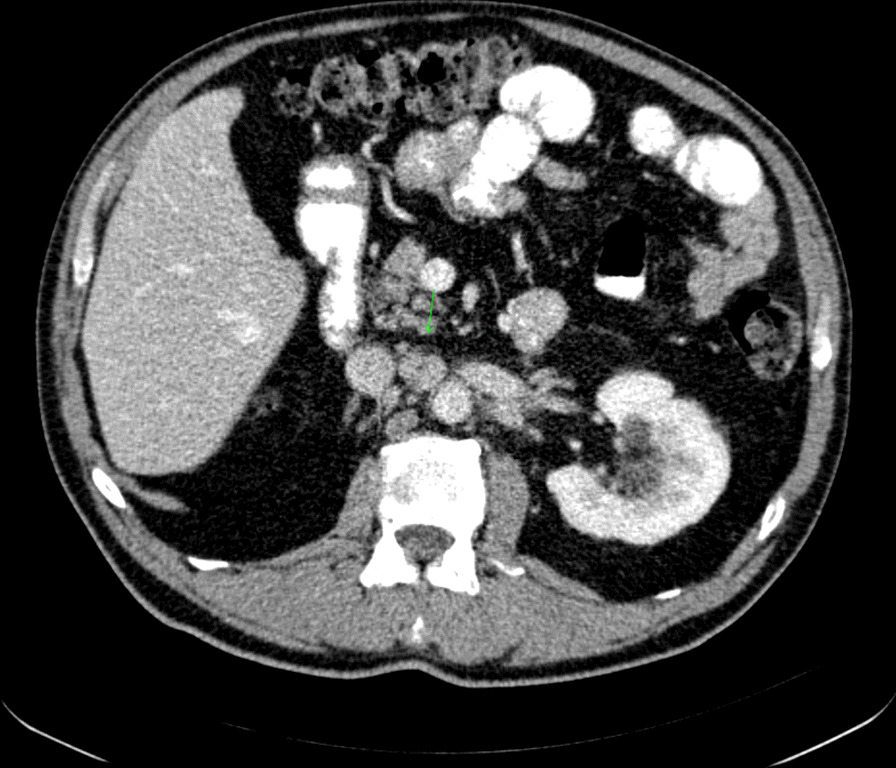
Computed tomography scan before atezolizumab treatment initiation (January 17, 2018): aorto-caval retroperitoneal lymph node—the target lesion (25 mm × 18 mm)—green arrow
